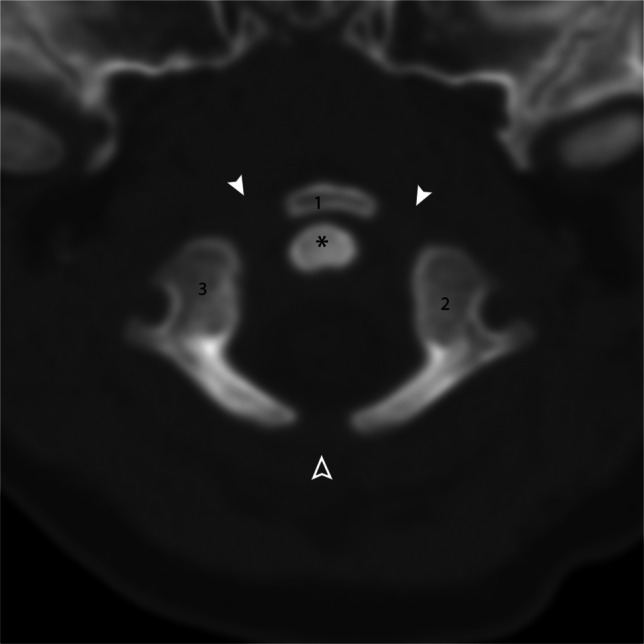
Axial CT of the C1 vertebral body of a 5-month-old male. C1 has three ossification centers: anterior arch (*1*) and paired neural arches (*2* and *3*). Three synchondroses, the paired neurocentral synchondroses (*arrowheads*) and the posterior synchondrosis (*hollow arrowhead*), are present
